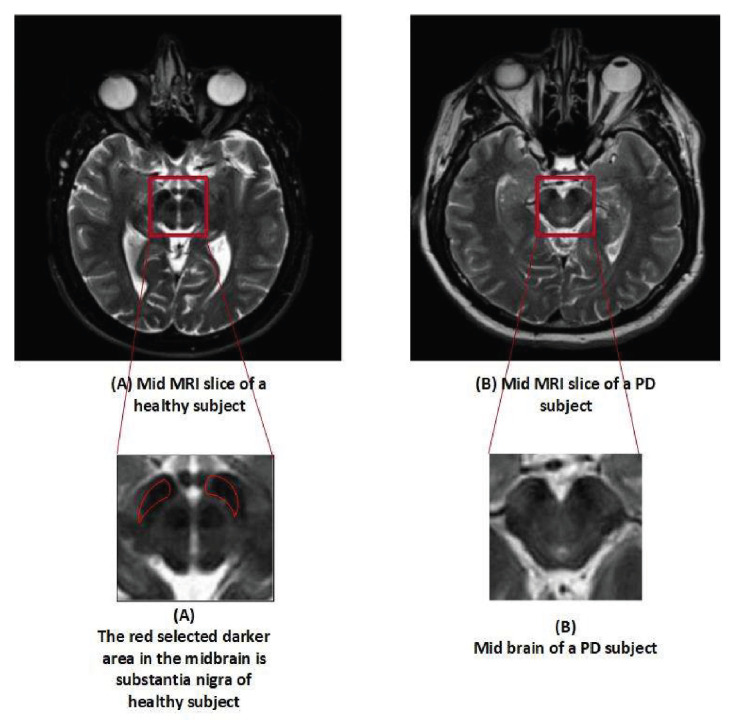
Slices of an MRI scan of an HC and PD patient.
